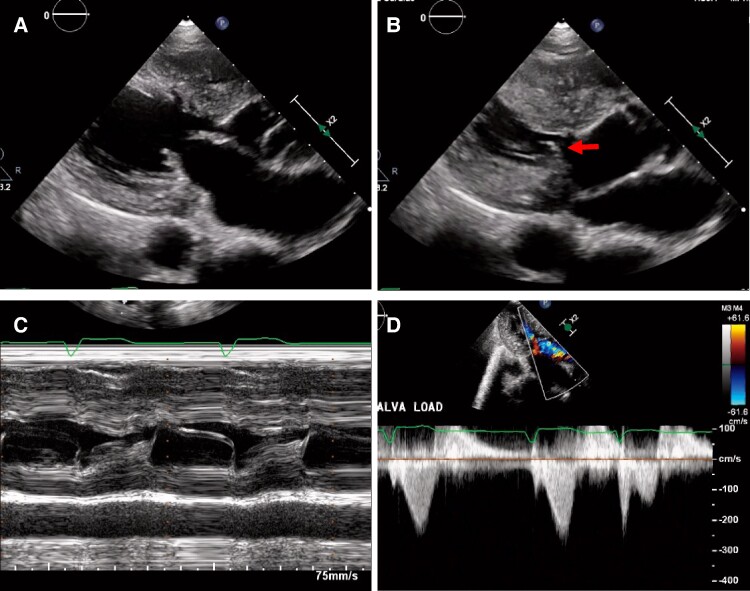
Echocardiography: (*A)* Mid-diastolic image shows hypertrophy of the left ventricular septum (16 mm) and a left ventricular non-compaction–like structure. *(B)* Systolic image shows the posterior mitral valve moving to contact the left ventricular septum (arrows). *(C)* M-mode image shows systolic anterior motion of the posterior mitral valve. *(D)* Doppler image shows left ventricular outflow tract obstruction of 31 mmHg by the Valsalva manoeuver.
